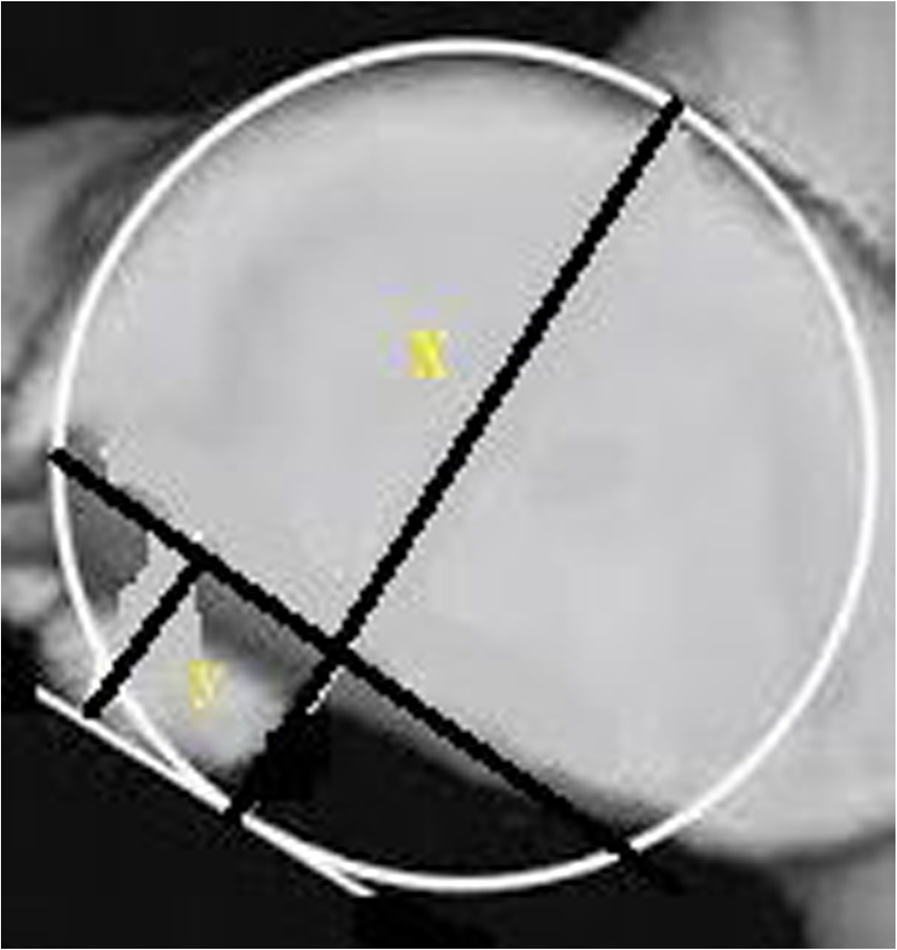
The Computed Tomography of the patient’s shoulder with the Bankart lesions. X: The inferior glenoid circle. Y: The eroded missing area. Images were checked by two engineers (diploma course in musculoskeletal imaging assessments in PR. China after MD radiology) trained in musculoskeletal imaging
